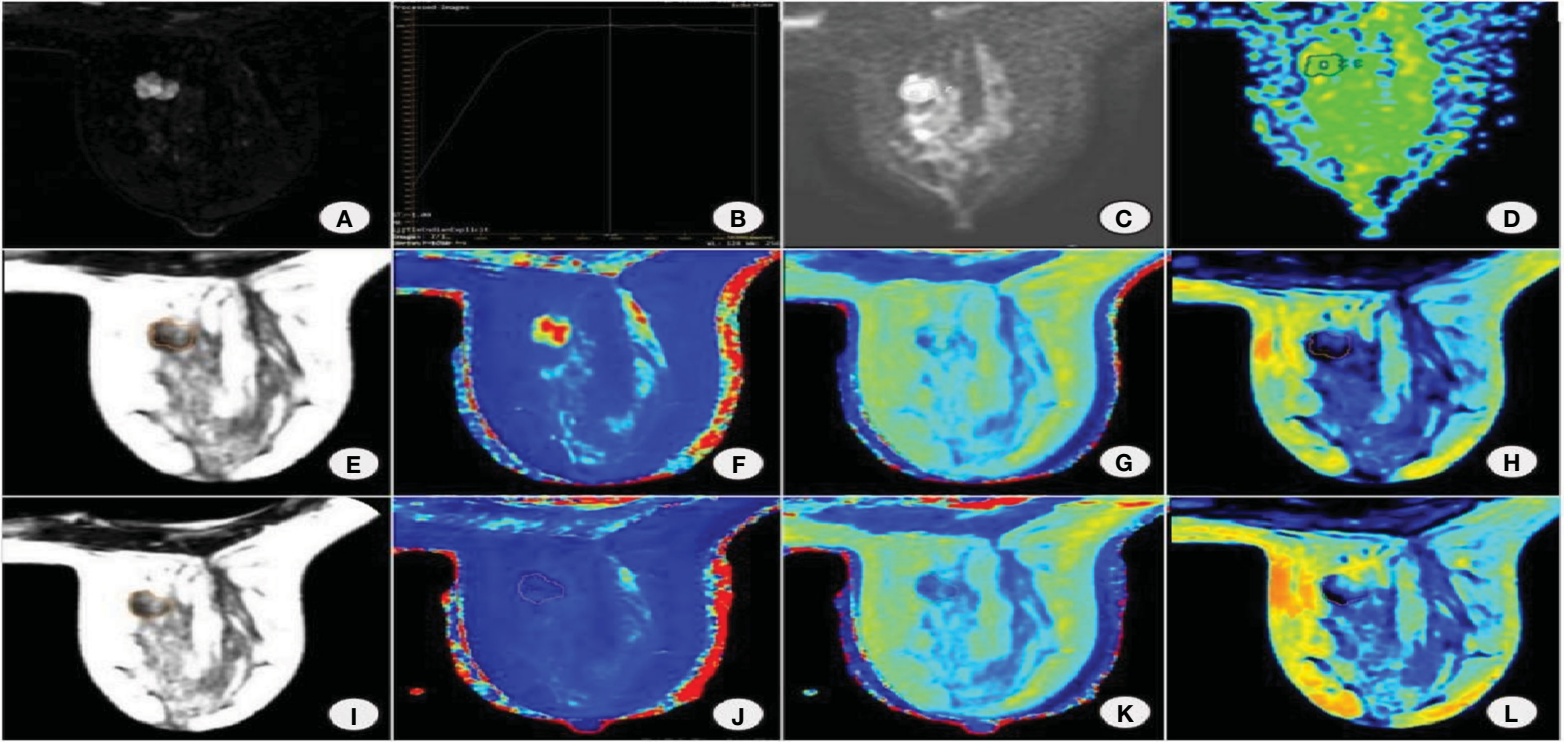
Female, 38 years old with right breast fibroadenoma. (A) DISCO enhancement image showing an irregular mass with irregular margin and uneven enhancement in the right breast inner quadrant. (B) TIC is a plateau. (C) DWI shows mass diffusion limitation. (D) ADC pseudocolor map. Image reconstruction with MAGiC. (E) SyMRI T2WI. (F) T1 map. (G) T2 map. (H) PD map. (I) SyMRI T2WI enhancement. (J) T1 enhancement map. (K) T2 enhancement map. (L) PD enhancement map.
